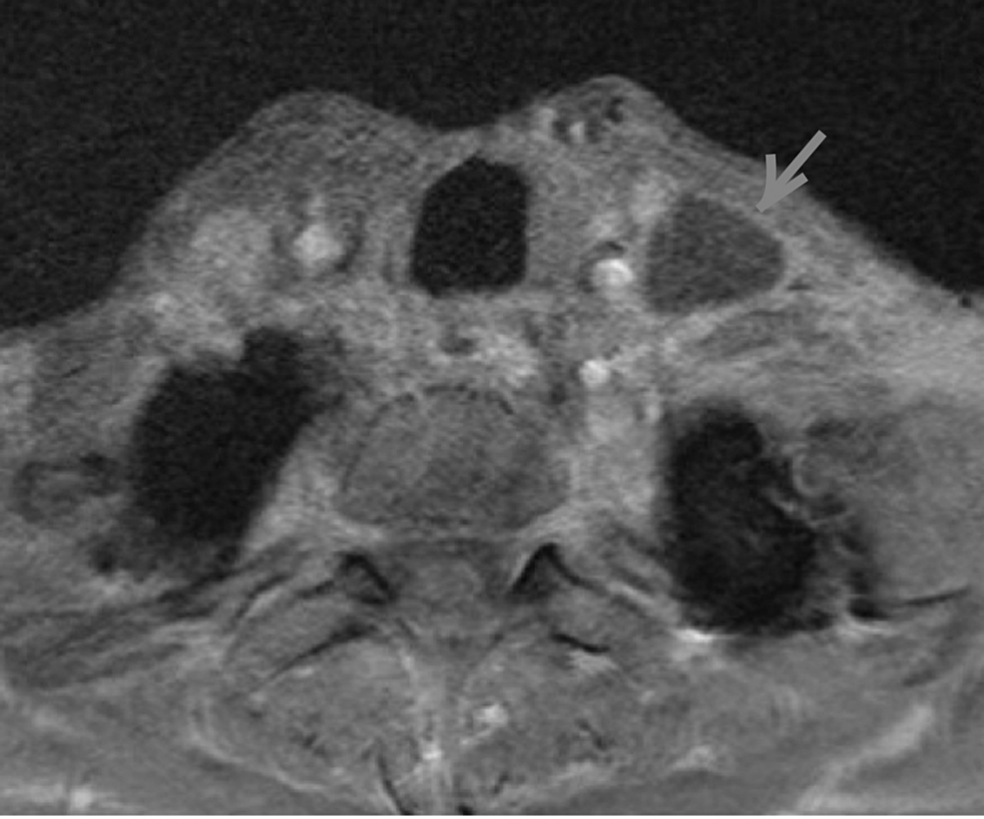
Arrow points to the chyloma on axial T1-weighted, gadolinium-enhanced MRI of the neck. The chyloma was located posterolateral to the left internal jugular vein at the level of the trachea (left level IV of the neck). At this location, the thoracic duct is expected to traverse the neck before it drains into the confluence of the internal jugular and subclavian veins. The chyloma was a 3.4 x 2.2 x 1.7cm circumscribed cystic lesion that was hypointense on T1 and did not enhance with the administration of gadolinium. The rest of the neck was unremarkable.
